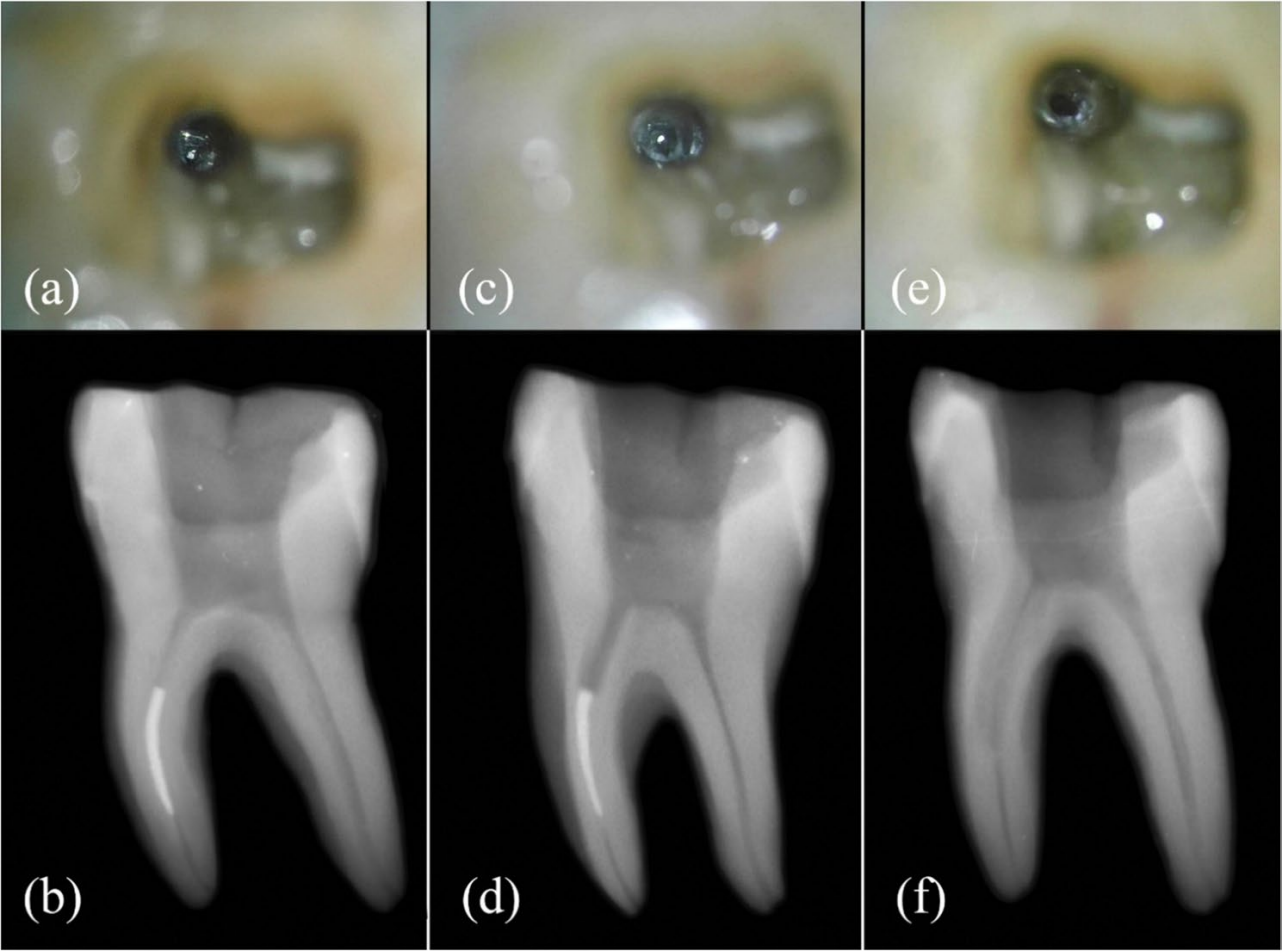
Conventional staging platform (a) Clinical photograph of separated instrument fragment separated in middle third of mesiobuccal canal under 11x magnification of DOM, (b) pre-operative radiograph, (c) conventional staging platform was created at 360° around instrument fragment, (d) intraoperative radiograph of the platform, (e) mesiobuccal canal after retrieval of the instrument fragment, (f) post-operative radiograph to confirm complete removal of the instrument fragment without any retained metal fragment
